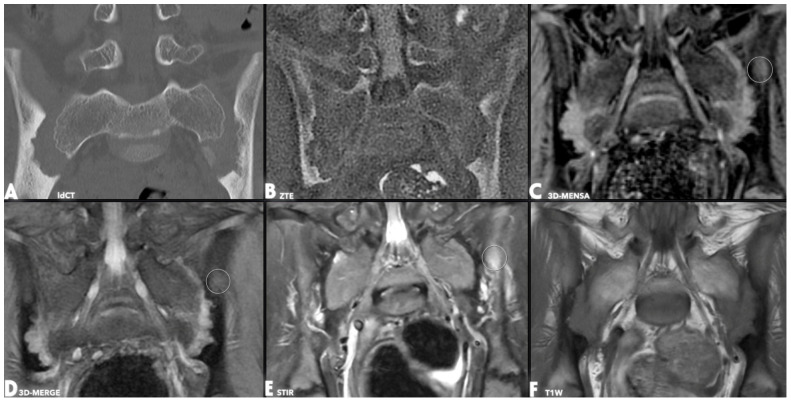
Coronal oblique images from the same slice and date in a 13-year-old patient comparing low-dose CT (A) and various MR sequences (B–F). Bilateral coalescent cortical erosions on the iliac sides and extensive adjacent subchondral sclerosis are observed. Subchondral sclerosis appears hyperdense on low-dose CT (A) and hyperintense on ZTE (B), whereas it is nearly signal-void on 3D-MERGE (D), 3D-MENSA (C), and T1-weighted (F) sequences. Erosions are sharply delineated on ZTE (B), 3D-MENSA (C), and 3D-MERGE (D) sequences, whereas they are visible but less detailed on the T1-weighted (F) image. A region of bone marrow edema in the left ilium is hyperintense on 3D-MENSA (C) with a signal comparable to STIR (E) and is also visible on 3D-MERGE (D) with lower hyperintensity (white circles).
